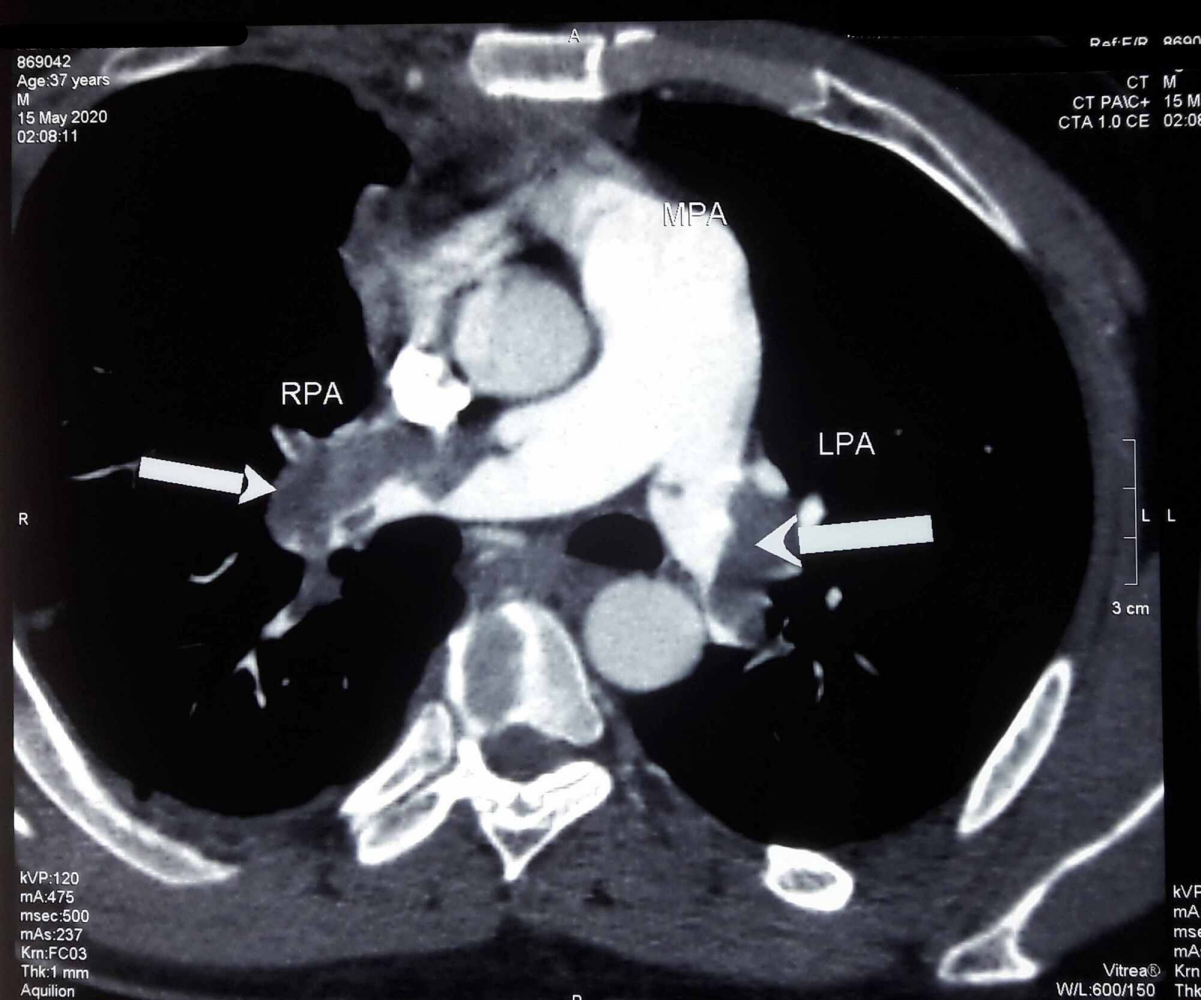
Computed tomographic pulmonary angiography. RPA (white arrow) - occlusive pulmonary thromboembolus in right pulmonary artery; LPA (white arrow) - occlusive pulmonary thromboembolus in left pulmonary artery.
